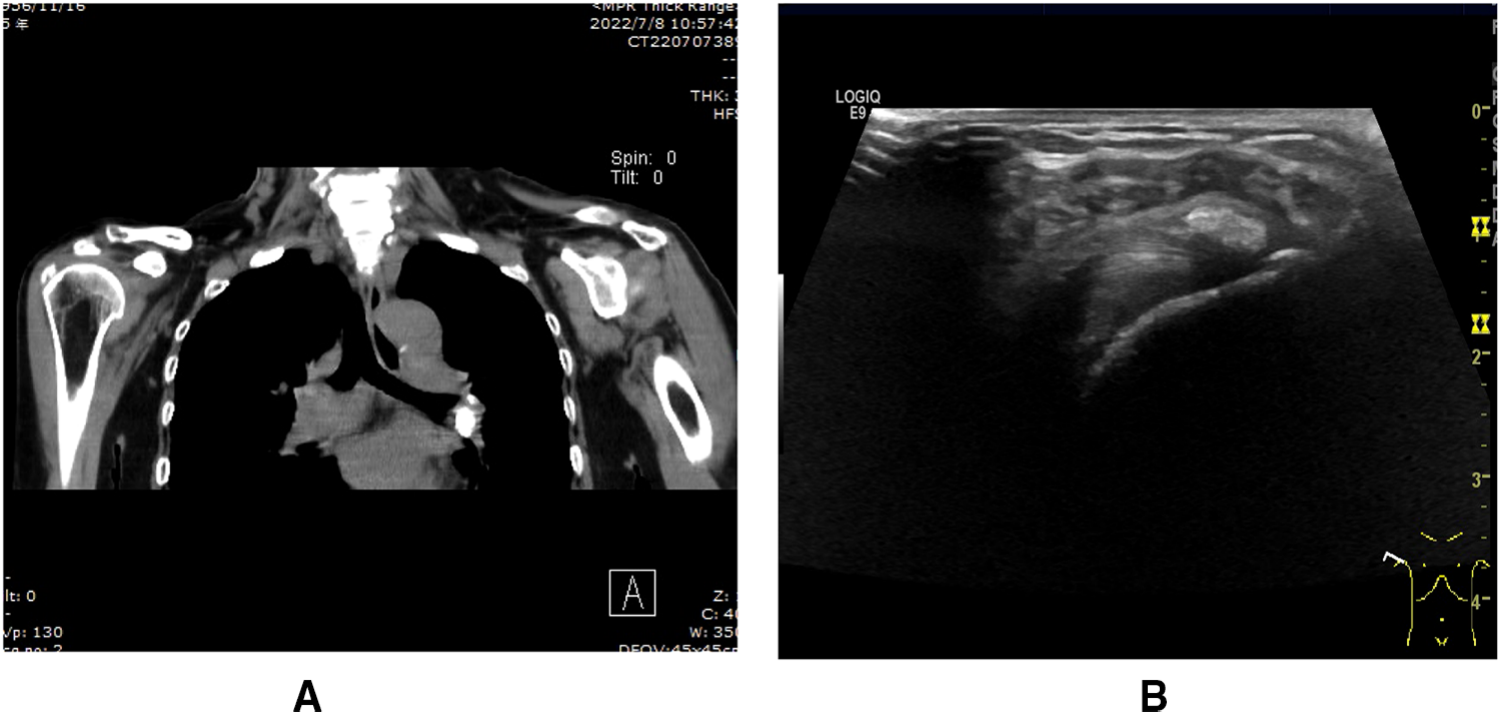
(A) Calcification foci of supraspinatus tendonitis on preoperative CT. (B) Calcific tendonitis calcification foci on preoperative shoulder. Ultrasound.
